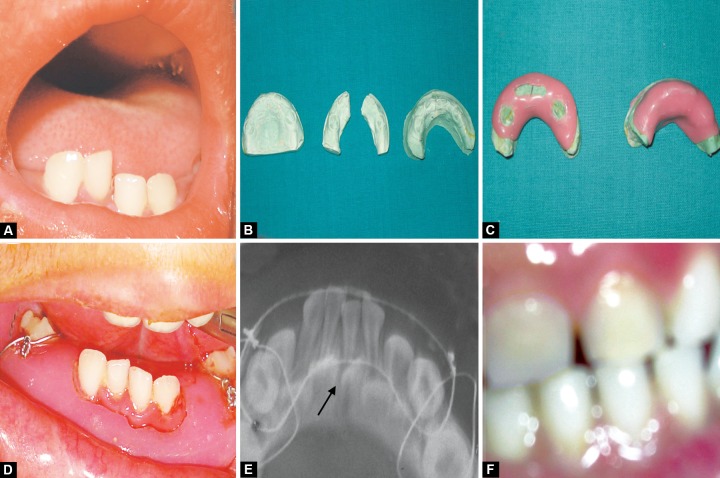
(A) Preoperative photograph, (B) fabrication of MacLennan splint, (C) fabricated MacLennan splint, (D) MacLennan splint with circummandibular wiring, (E) postoperative radiograph and (F) follow-up
